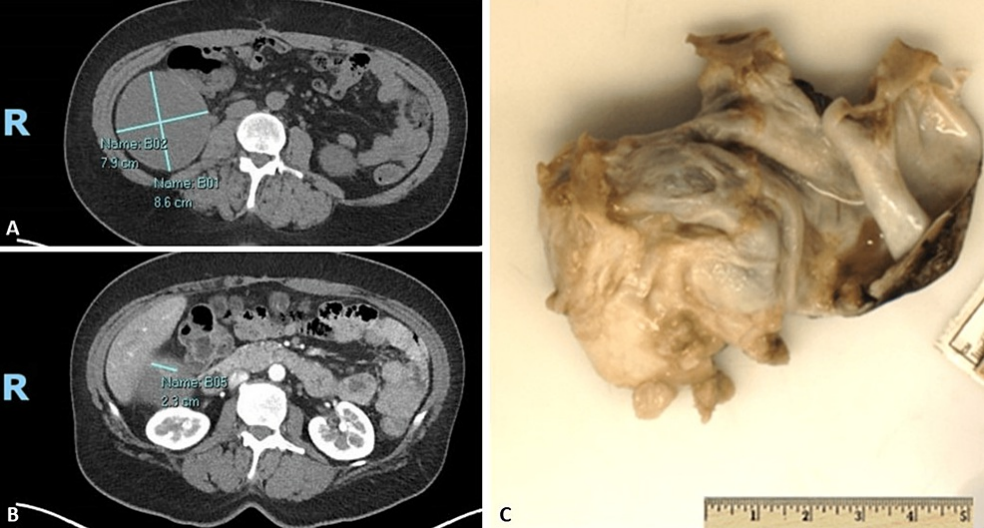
CT of the Abdomen and Gross Findings A: CT of the abdomen with contrast demonstrating an 8.6 × 7.9 cm mass at the anterior lower pole of the right kidney. B: CT of the abdomen with contrast demonstrating the largest mural nodule within the mass measuring 2.3 cm. C: Gross appearance of the unilocular cyst after evacuation of cyst contents. CT: computed tomography
